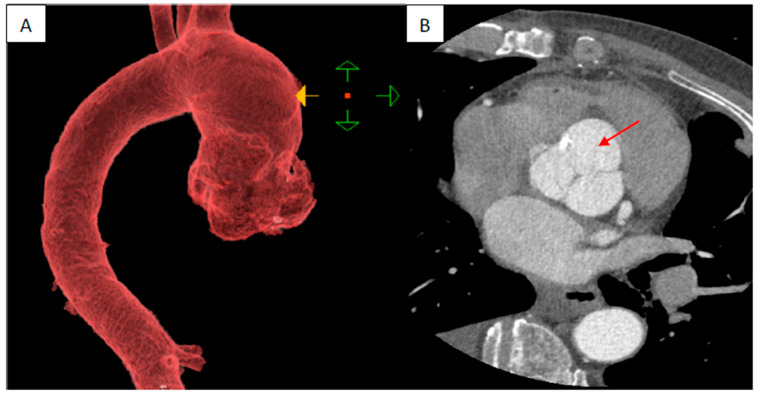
Preoperative contrast chest CT. (A) Reconstruction of ascending aorta and root. (B) The transverse plane at the level of the aortic sinuses shows an enlarged aortic root with the predominance of non-coronary sinus dilatation (arrow).
